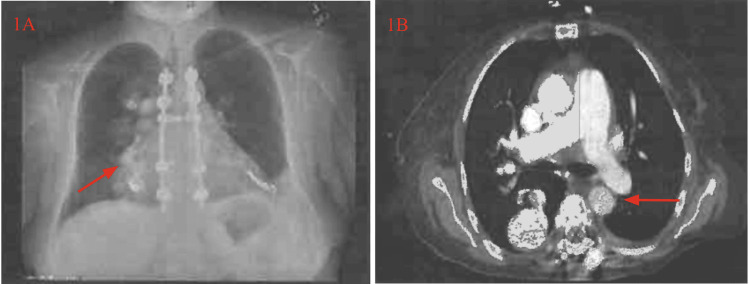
Chest X-ray and CT angiography demonstrating a right pulmonary arteriovenous malformation. Figure A shows a chest X-ray with a right perihilar opacity consistent with a pulmonary arteriovenous malformation (AVM). Figure B shows a CT angiogram confirming a large right-sided pulmonary AVM, which may contribute to increased cardiopulmonary demand in the setting of severe anemia. Source: Figure synthesized by MS and JRP with images extracted from the patient's scans.
